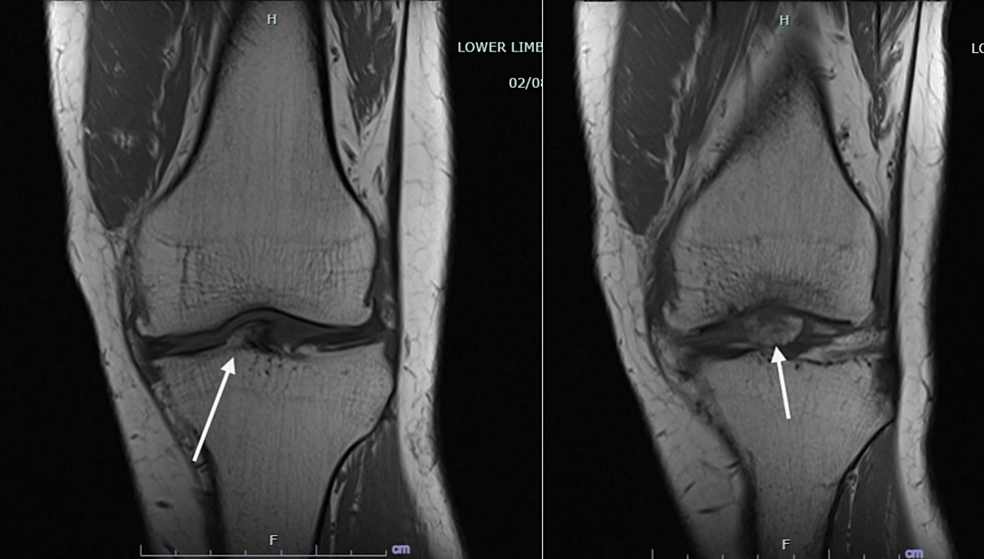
Coronal view of the MRI, demonstrating the presence of tibial osteophyte and loose body
